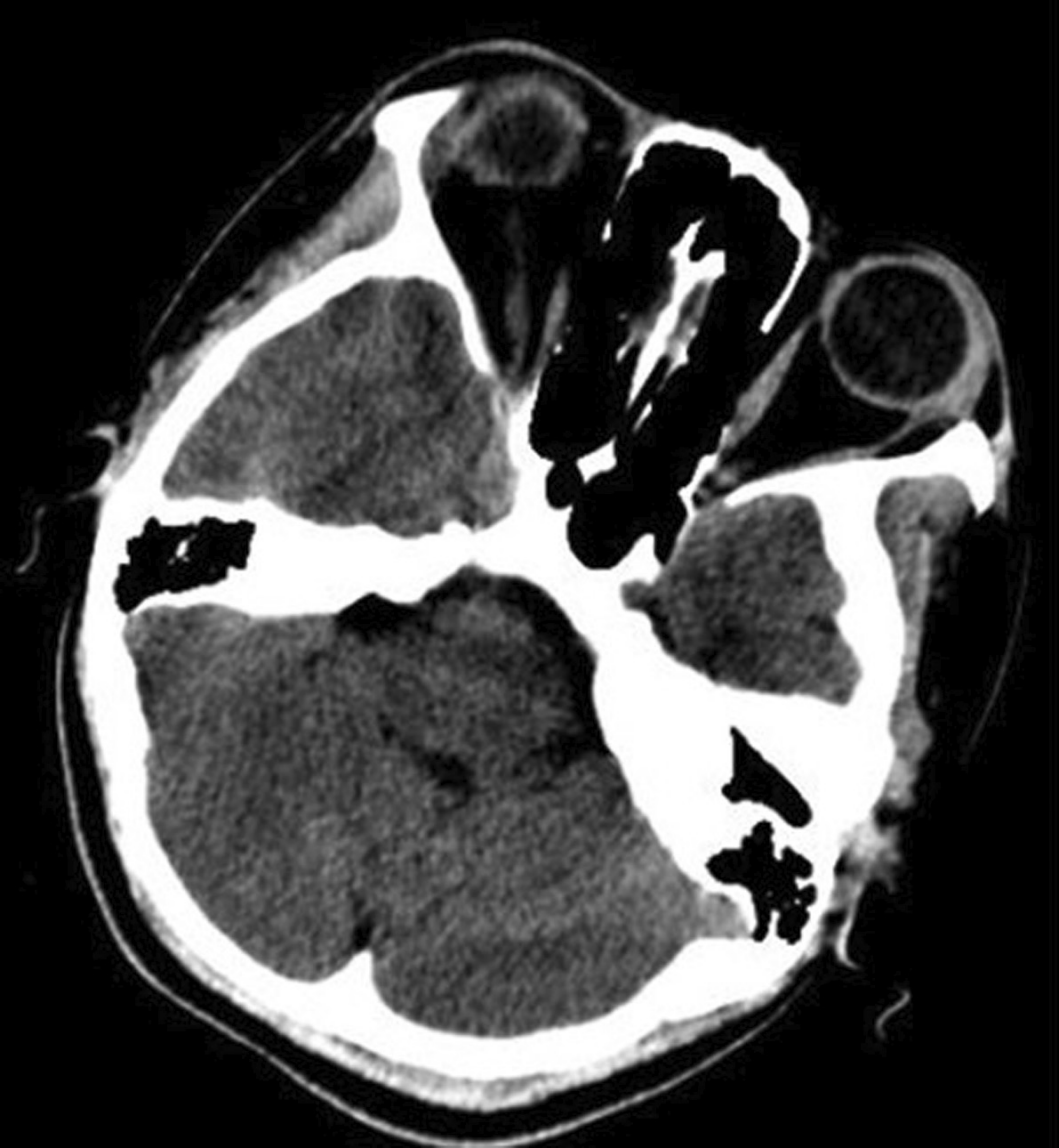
Oculocerebral CT scan with parenchymal window: regression of the intraorbital hematoma
